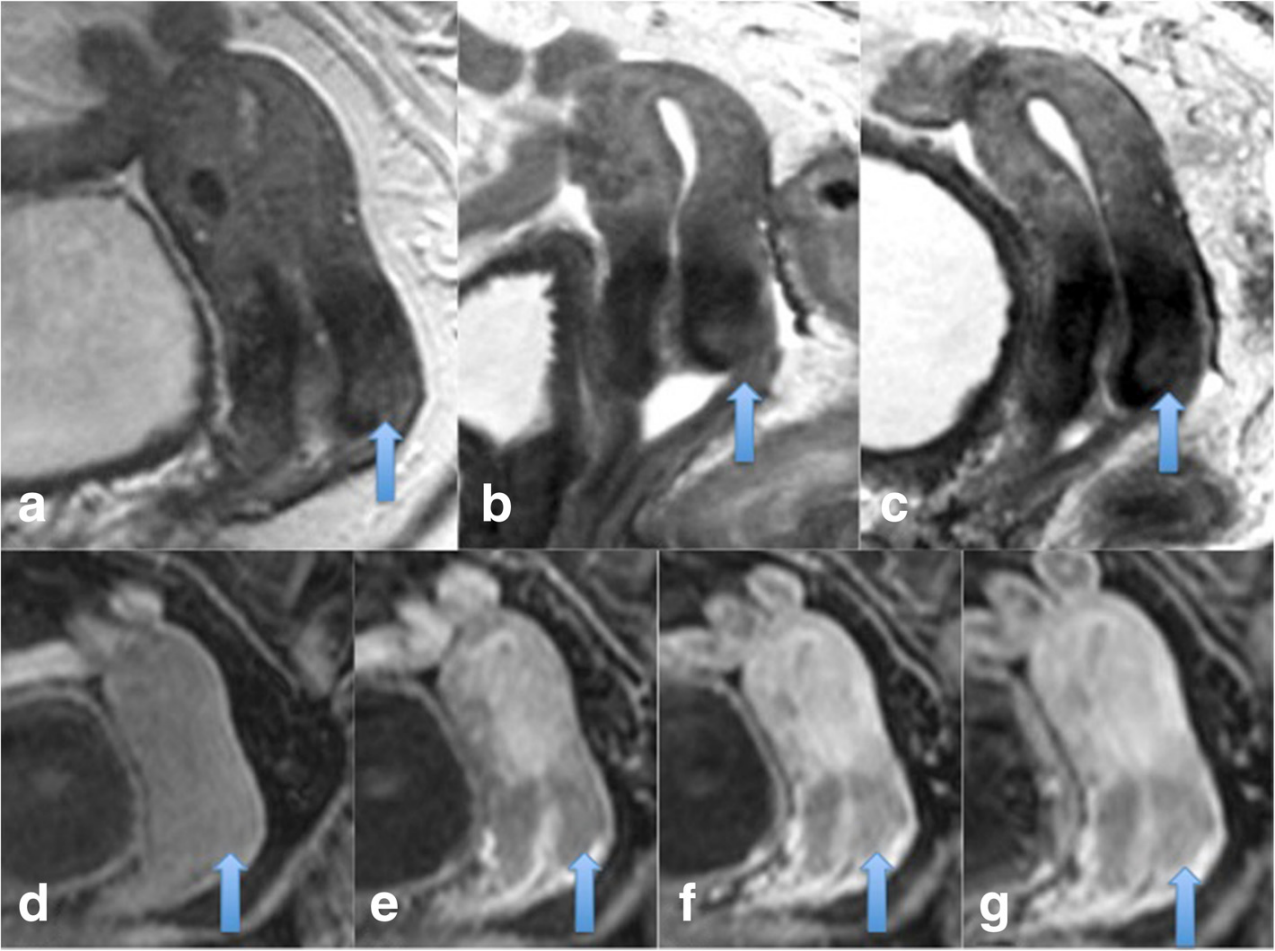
Poorly enhancing cervical tumour indicating poor response to treatment. Pre-treatment sagittal T2 MRI showing a tumour in the posterior cervical lip (a), at the end of treatment there is recurrent disease (b) and 6 months later residual disease is present (c). The pre-treatment DCE images are shown in figures d-g from pre-contrast to later contrast enhancement, minimal enhancement of the tumour is seen predicting the poor outcome to treatment observed.
